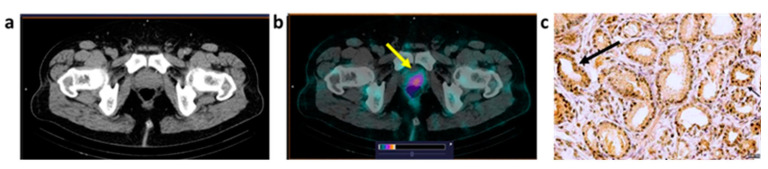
CT (a) and fused SPECT/CT (b) images of PCa patient (P1, adenocarcinoma) 2 h after injection of [^99m^Tc]Tc-maSSS-PEG_2_-RM26. A focus of increased [^99m^Tc]Tc-maSSS-PEG_2_-RM26 uptake (SUVmax = 1.21) is visualized in the prostate (yellow arrow). The upper setting of the scale window (24% of the maximum number) was adjusted to visualize the lesion. Moderate (2+) GRPR expression in adenocarcinoma cells (c) was detected by IHC analysis in tumor material (black arrow). Magnification 40×.
